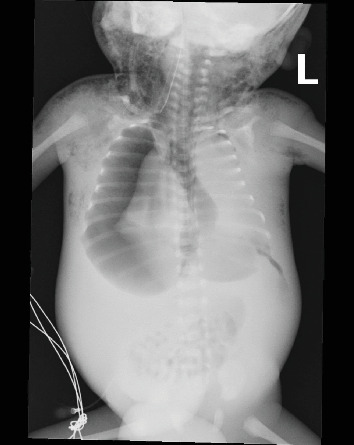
Chest radiograph frontal view. Large right pneumothorax, pneumomediastinum, and surgical emphysema in the neck and chest. Abnormally oriented/positioned endotracheal tube. The radiograph also showed nonaerated lungs, inverted diaphragm, abdominal distension, and thickened subcutaneous soft tissues.
